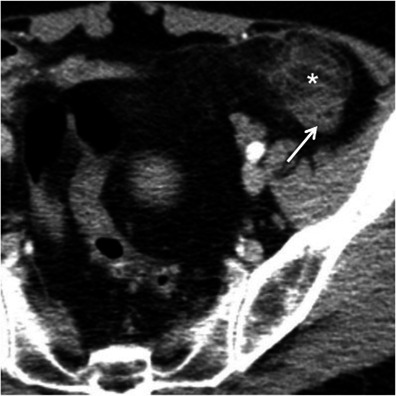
Epiploic appendagitis of the descending colon. Axial contrast-enhanced CT scan shows the inflamed epiploic appendage anterior to the colonic wall (*asterisk*) with adjacent disproportionate fat stranding and minimal wall thickening of the descending colon (*arrow*). *Adapted from the electronic poster “Bowel wall thickening—a complex subject made simple” DOI:*10.5444/esgar2011/EE-063
